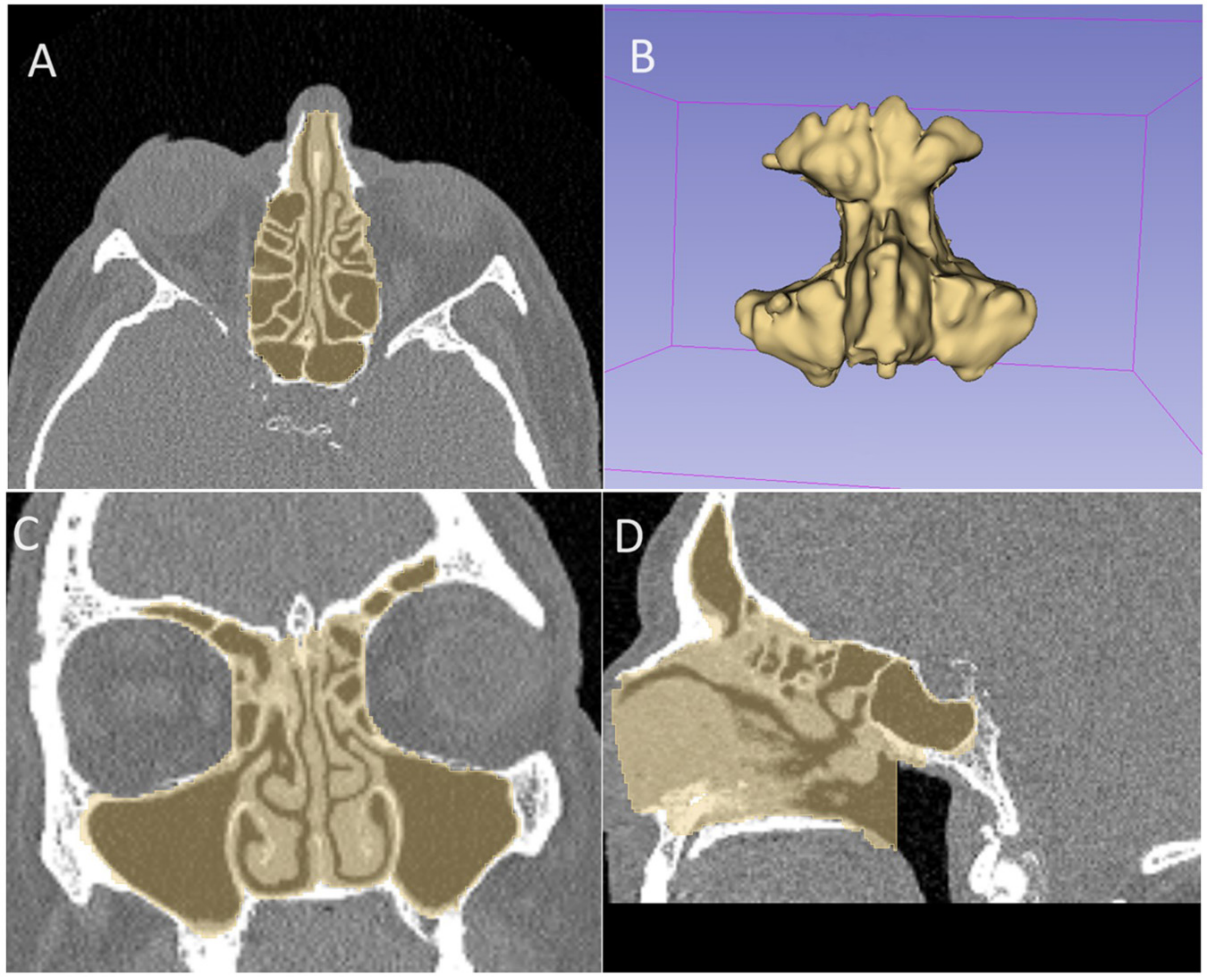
Sample segmentation of the paranasal sinuses for radiomic analysis. Screenshot from 3-dimensional (3D) Slicer (Open Source, http://www.slicer.org) application demonstrating a sample manual segmentation of the paranasal sinuses on computed tomography (CT) imaging, with axial views (A), 3D segmentation of the paranasal sinuses (B), coronal views (C), and sagittal views (D).
